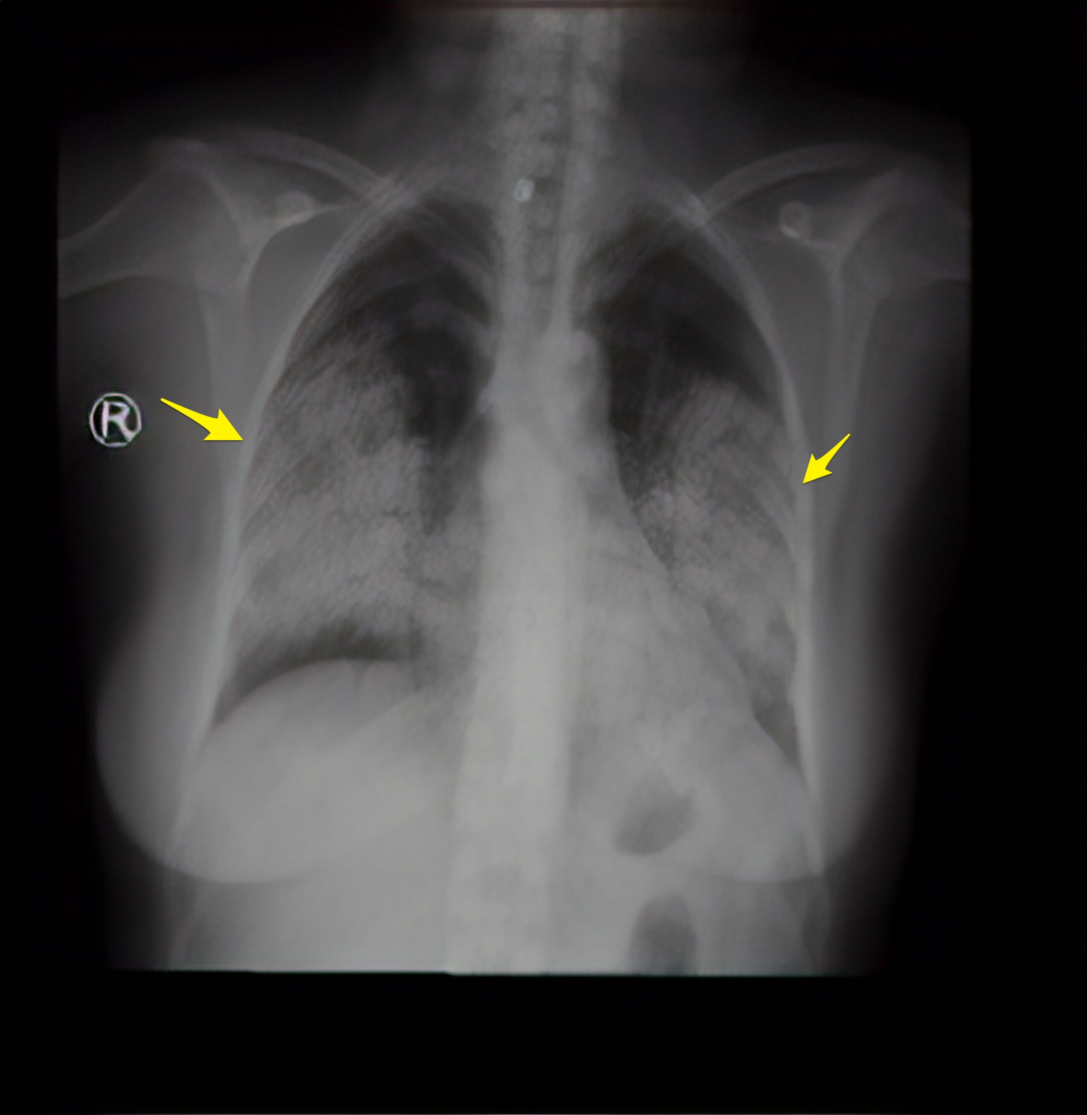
Chest X-ray (PA view) Posteroanterior (PA) view: diffuse bilateral middle and lower zone heterogeneous opacity (Yellow arrows).
